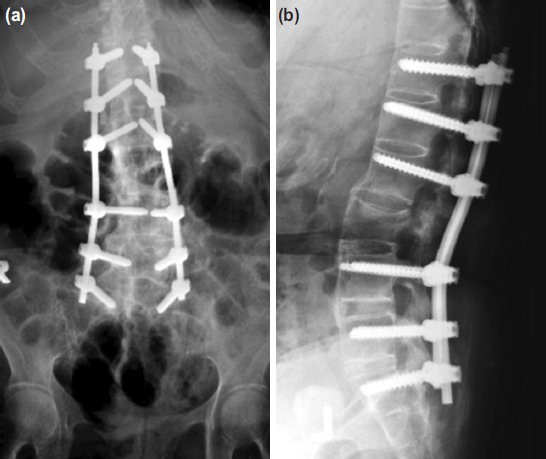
(a) Immediate postoperative AP and (b) lateral plain radiographs of the same patient treated with MISt with percutaneous pedicle screws from T12 to S1.
